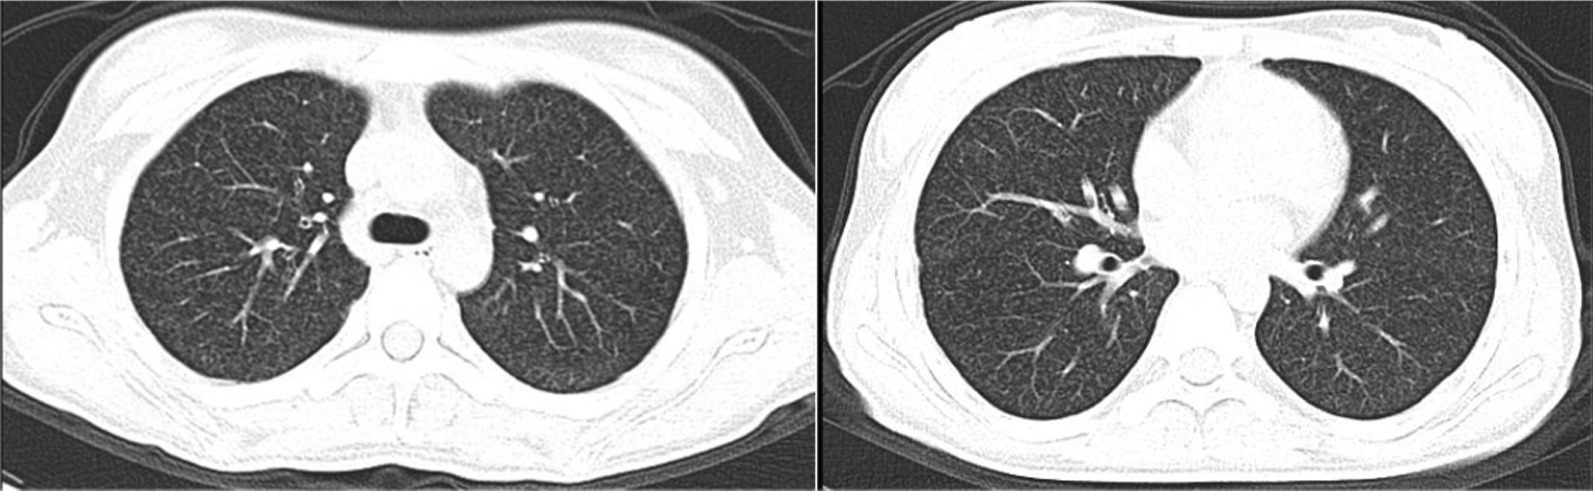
Representative slices of chest CT images showed diffuse miliary nodules in both lungs
